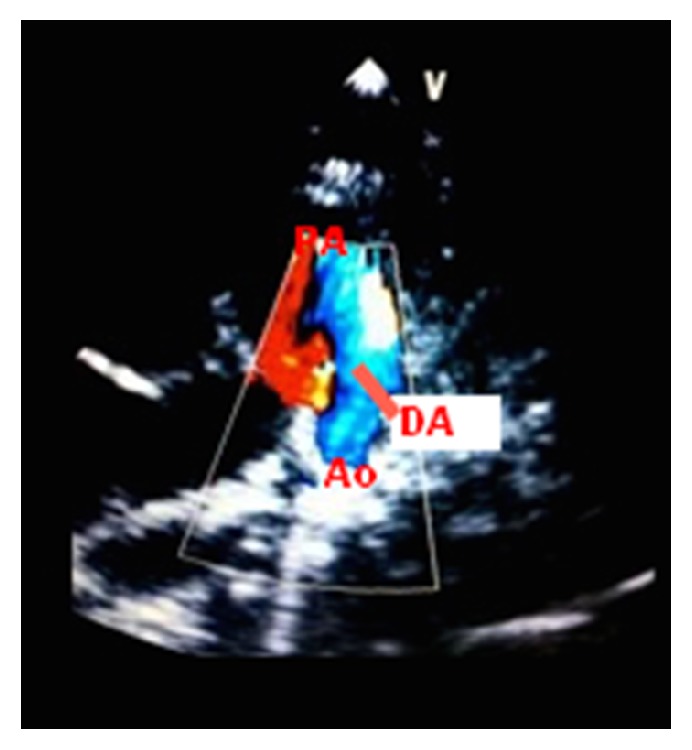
Transthoracic echocardiogram showing patent ductus arteriosus with flow (blue) from the pulmonary artery to the aorta. PA: pulmonary artery, DA: ductus arteriosus, and Ao: aorta.
